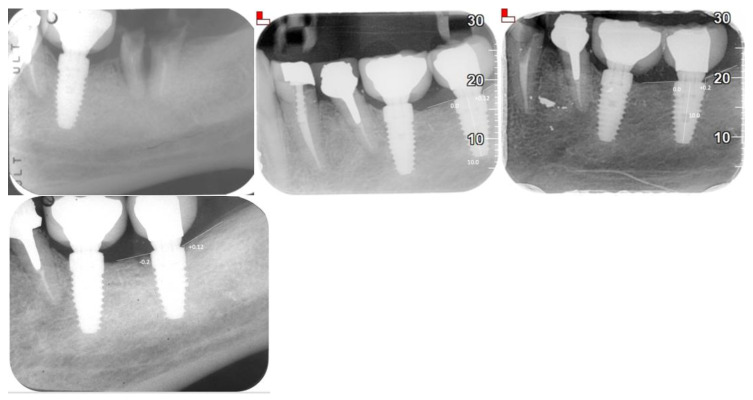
X-ray follow-up during the time. Extractions from 2021, 2022, and 2024.
